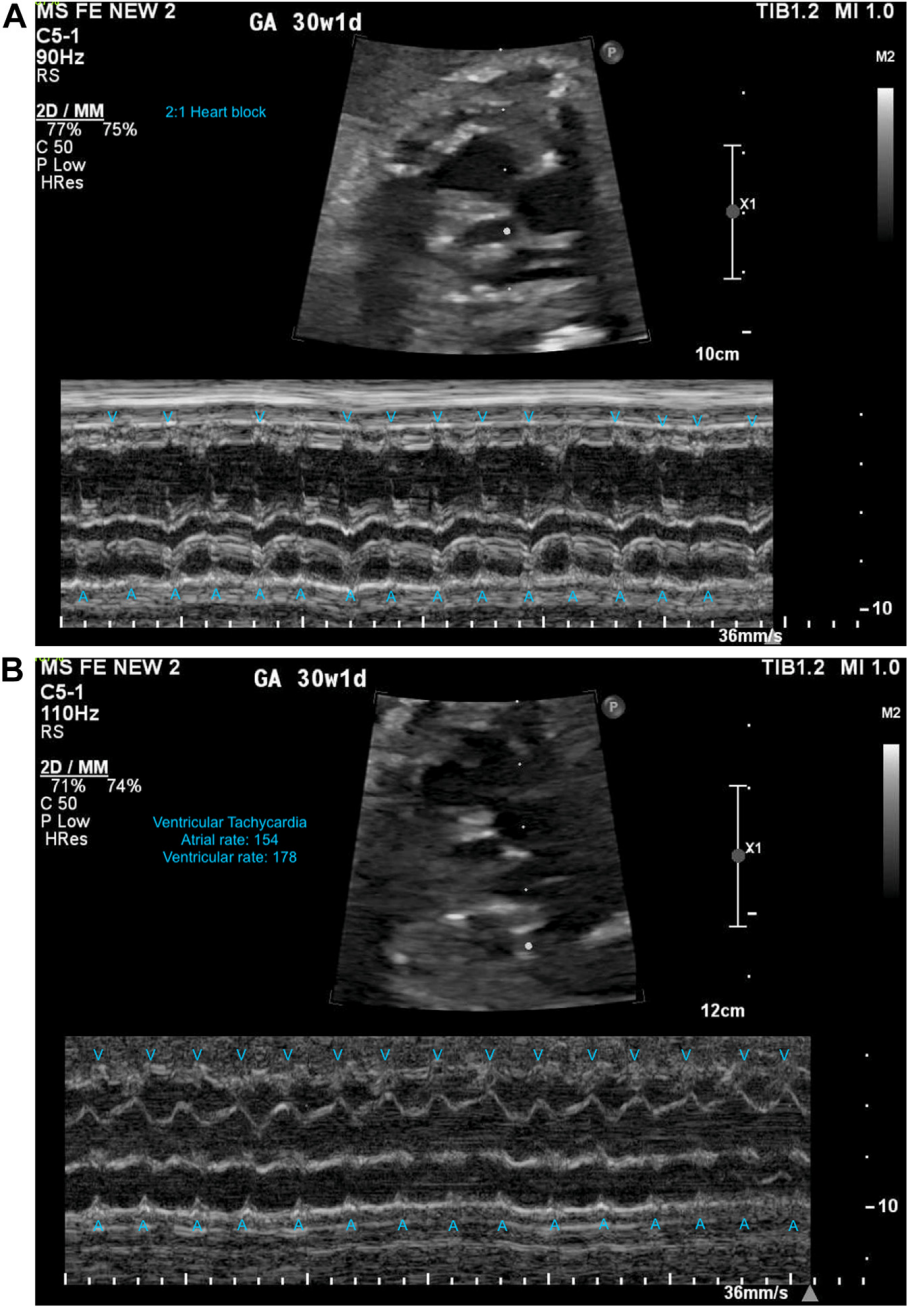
Fetal Echocardiogram at 30 Weeks 1 Day M-mode echocardiogram annotated with ventricular (V) and atrial (A) contractions. (A) Episodes of 2:1 atrioventricular block. (B) Ventricular tachycardia. GA = gestational age.
